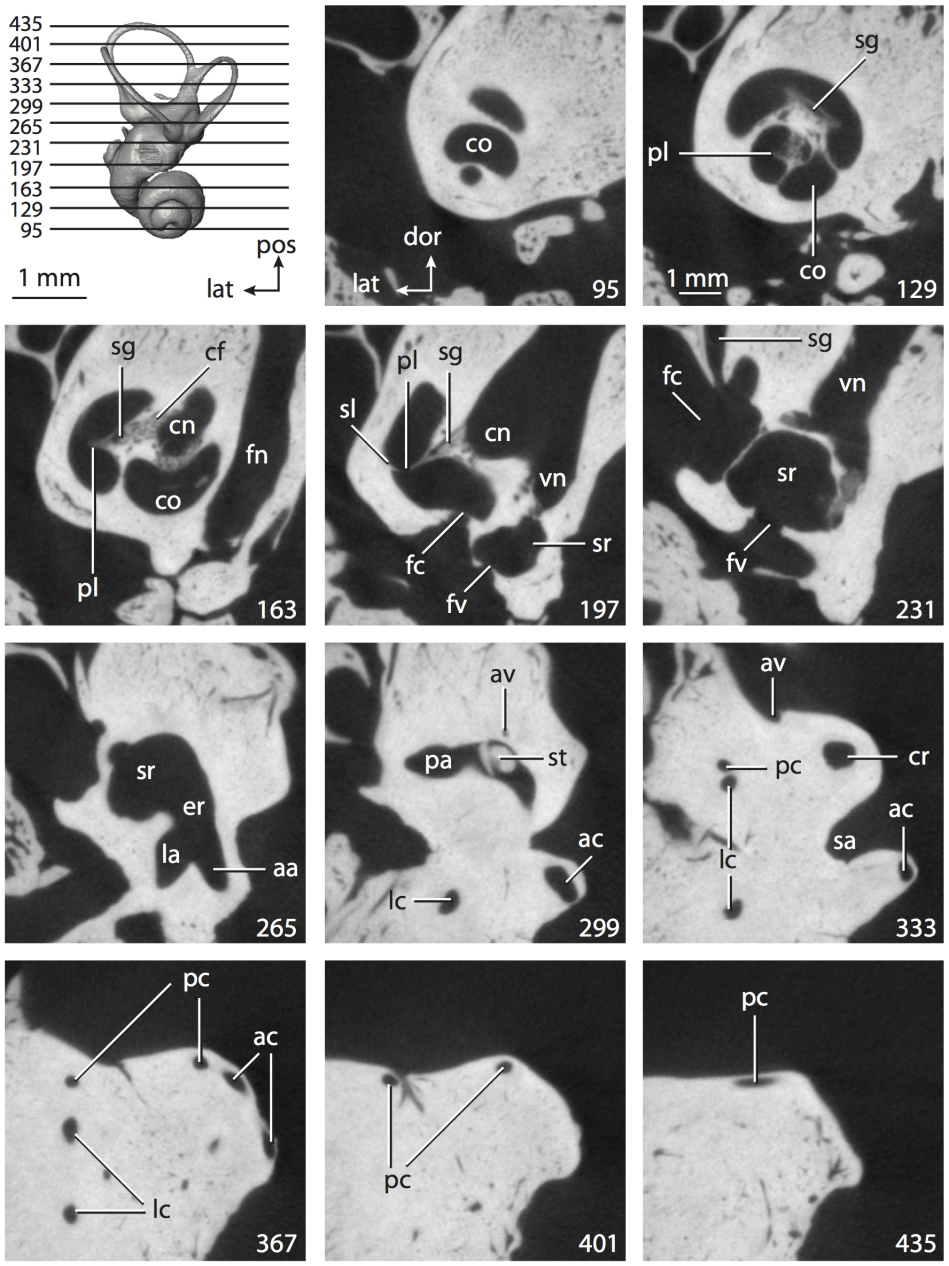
CT slices through ear region of*Eumetopias jubatus*. Abbreviations listed at the end of the Materials and Methods section.
